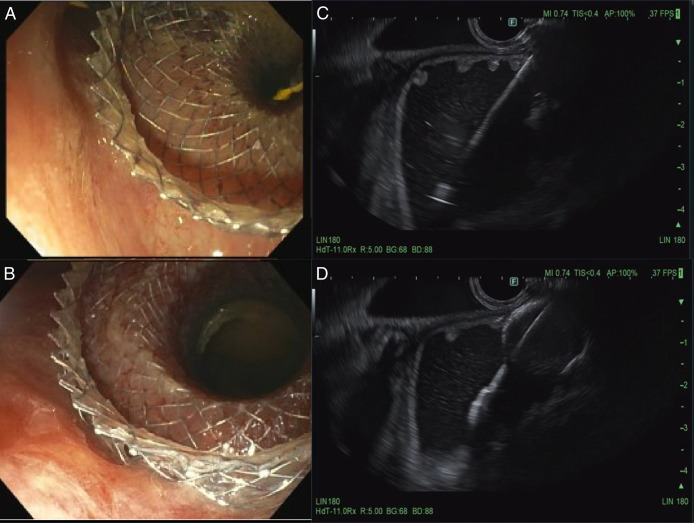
(A, B) Endoscopic images of an Axios stent deployed from the distal descending colon toward likely mid-jejunum, (C) EUS image before stent deployment (endoscopy within the descending colon lumen, which is seen above the jejunum lumen), and (D) EUS image after stent deployment. EUS, endoscopic ultrasound.
